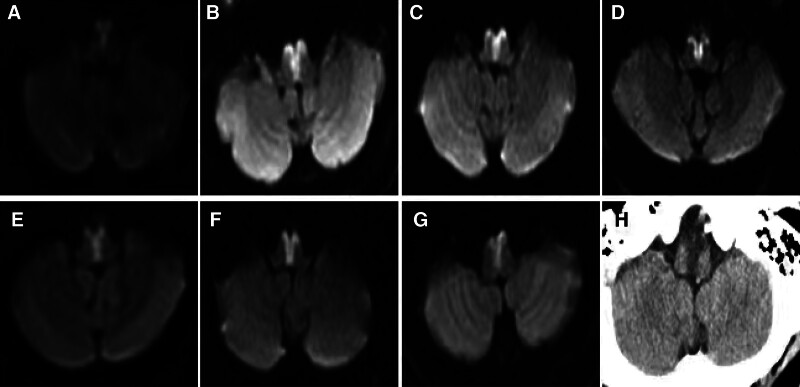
(A–G) Imaging characteristics of the bilateral medial medullary infarction. MRI of the brain using DWI revealed a characteristic high-intensity signal in the bilateral medial medulla oblongata, which often appears as a “heart appearance,” “V” appearance, or “Y” appearance. (H) CT scan revealed “Y” appearance low density image of the medial medulla oblongata. DWI = diffusion-weighted imaging, MRI = magnetic resonance imaging.
